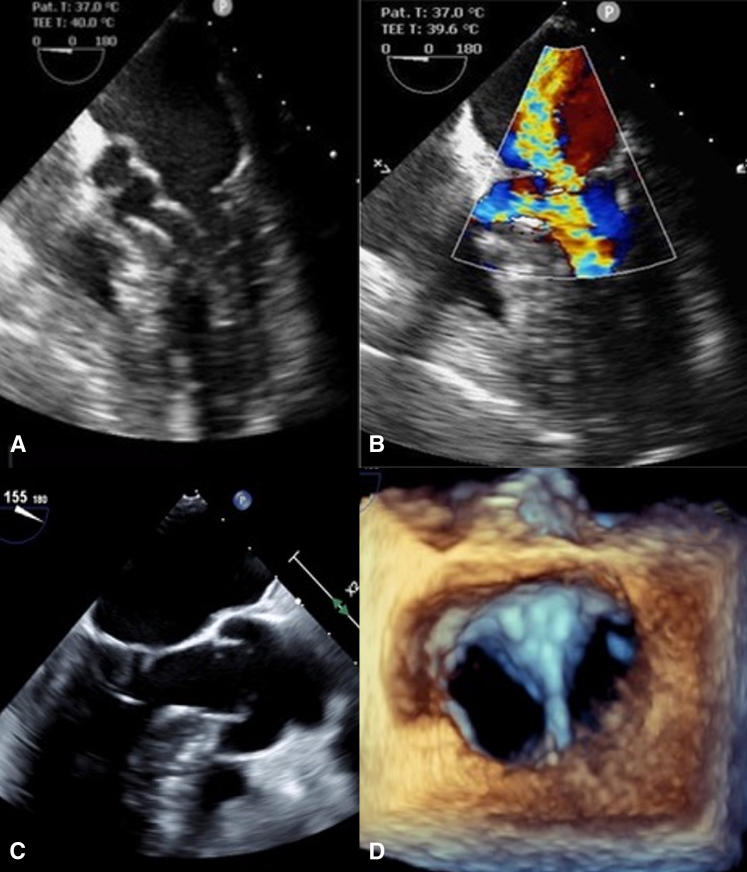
Preoperative transesophageal echocardiogram showed left ventricular outflow tract (*LVOT*) obstruction with systolic anterior motion (*SAM*) (A) and moderate mitral regurgitation (B). Postoperative imaging demonstrated resolution of LVOT obstruction and disappearance of SAM (C) after placement of an Alfieri stitch between A2 and P2 (D).
